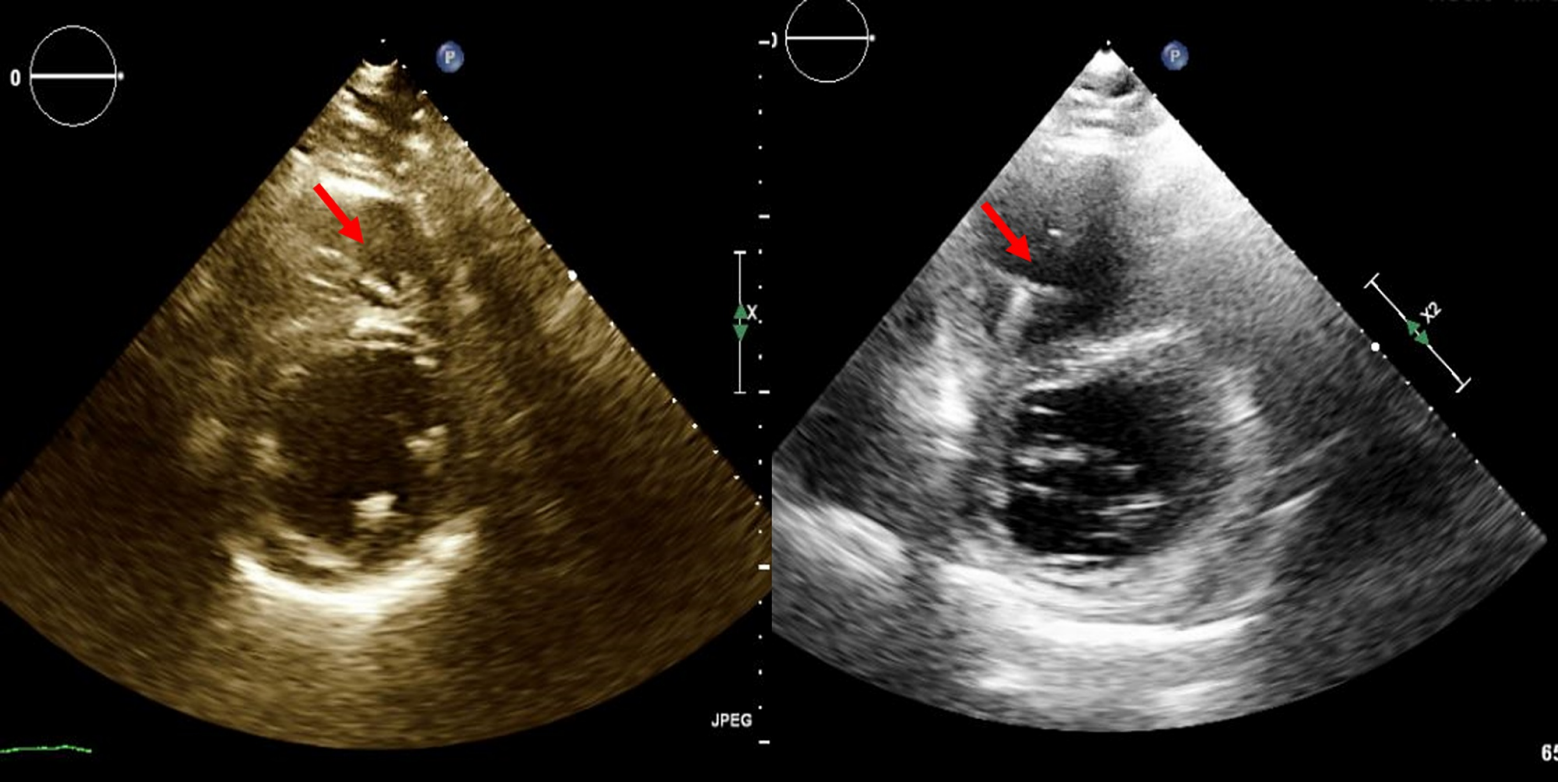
Transthoracic echocardiogram before (left) and after AAA repair (right), showing right ventricular dilation and flattening of the interventricular septum. AAA: abdominal aortic aneurysm
